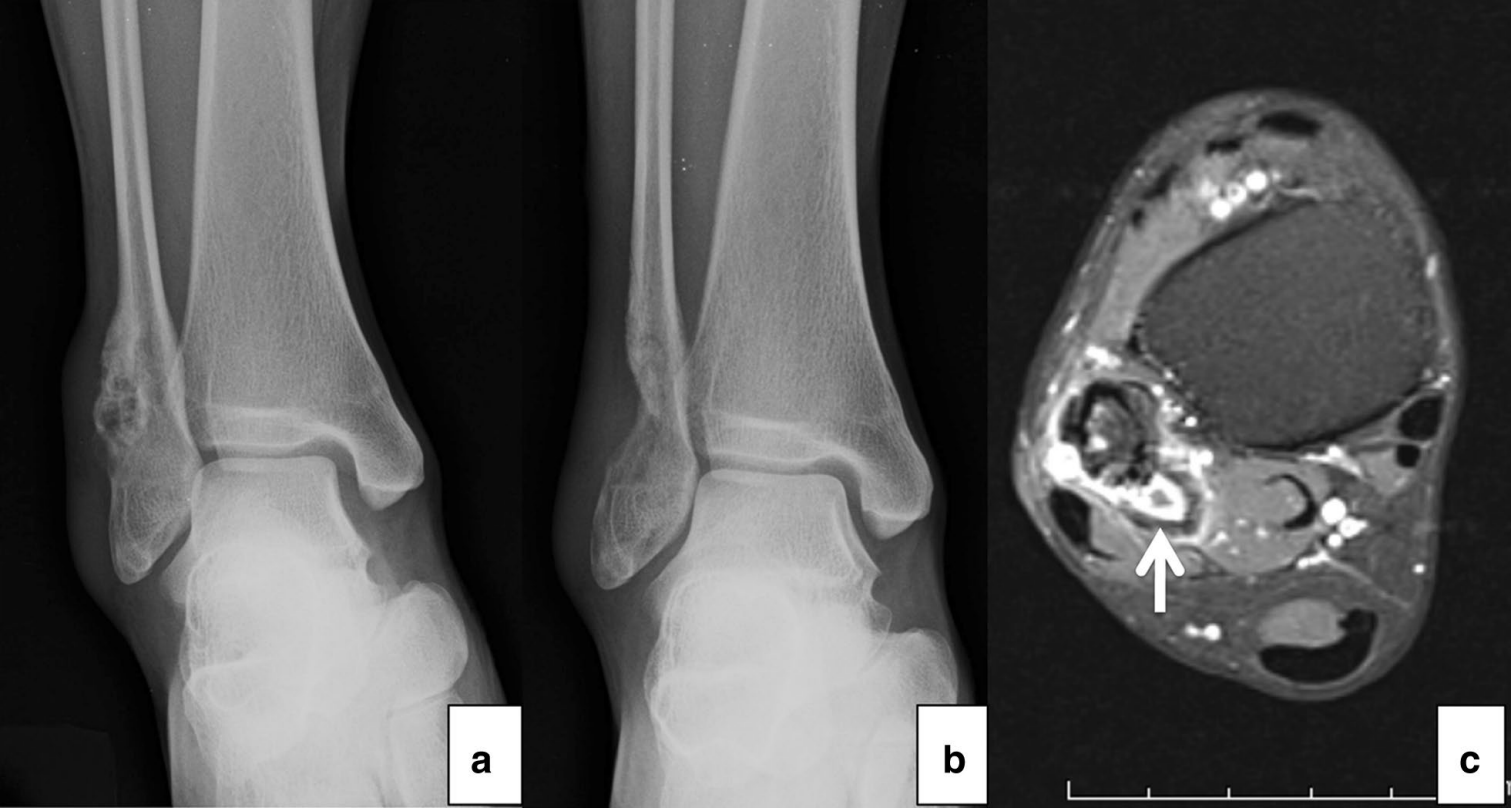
The initial radiograph showing an eccentric osteolytic lesion in the distal fibula (a) and post incisional biopsy (b). Axial magnetic resonance imaging (post intravenous gadolinium T1-weighted image with fat suppression) demonstrating a recurrent tumor near the biopsy site (arrow) (c)
